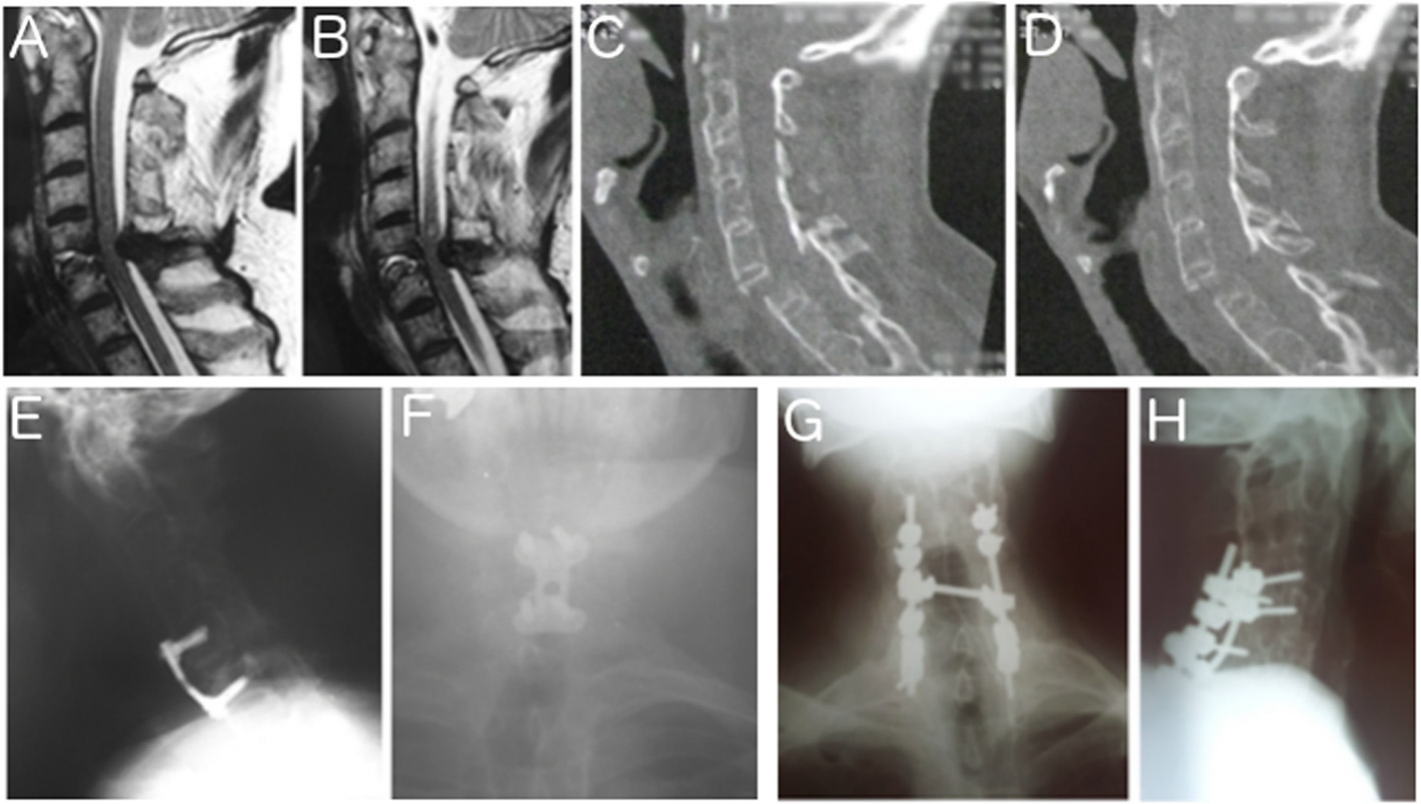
Pre and postoperative images of Patients 1 and 2. Observe in (a) and (b) the fracture-dislocation of cervical C5 to C6 in the initial sagittal magnetic resonance imaging (T2-weighted) of Patient 1. In (c) and (d) it is possible to see a fracture-dislocation of C5 to C6 with disruption of anterior and posterior complex in the sagittal computed tomography scan images of Patient 2. e Demonstrates the postoperative radiography in lateral view and (f) the anteroposterior image demonstrating the short anterior fixation of C5 to C6; note the posterior decompression and stabilization performed in Patient 2 in the anteroposterior (g) and lateral (h) view of the radiographic images
